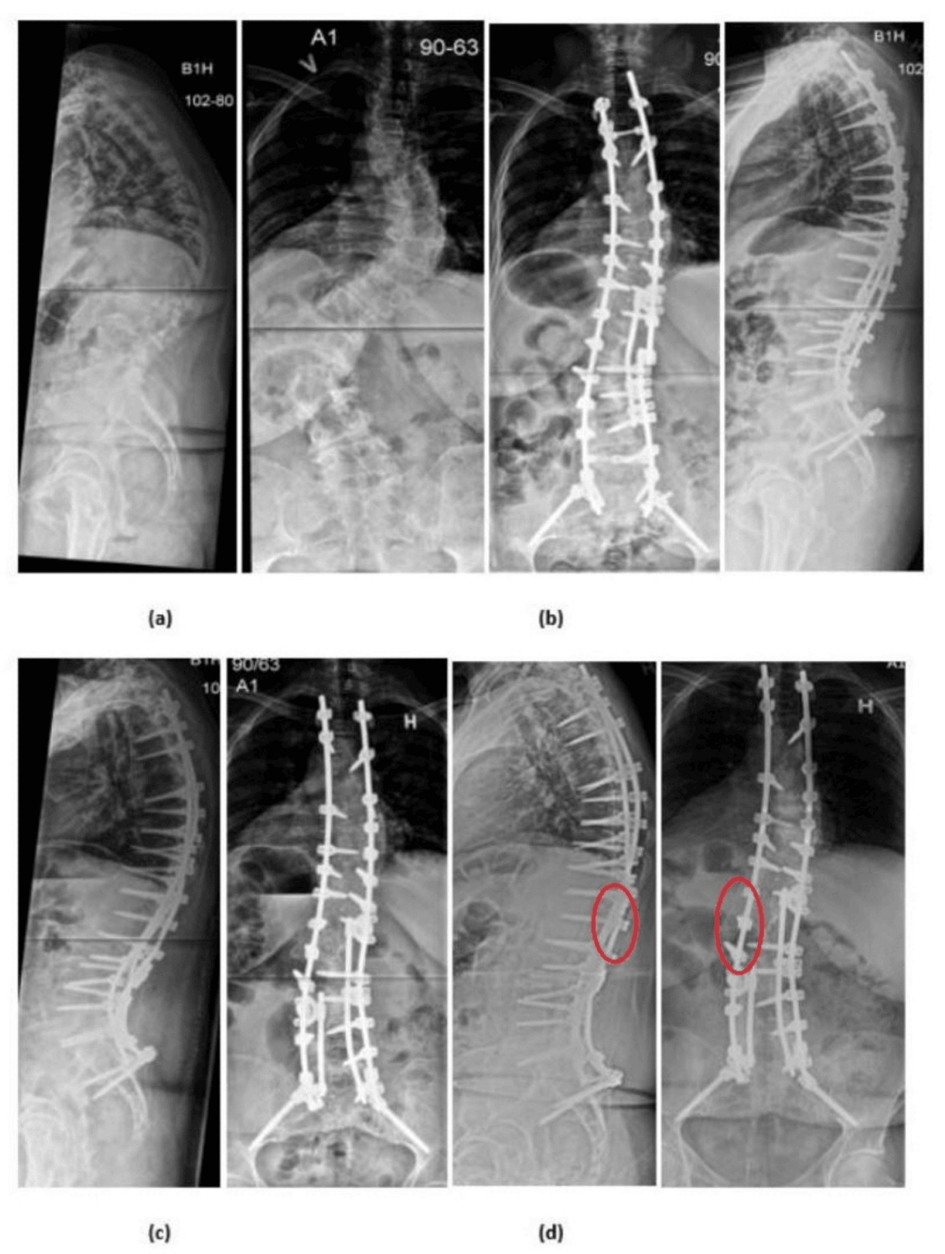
Case 2 A 53-year-old female was referred due to untreated thoracolumbar deformity known since childhood and progressing low back pain. She presented with kyphoscoliosis and a subjective feeling of positive sagittal imbalance (a). She underwent posterior instrumentation from Th2 to the pelvis with no supplemental ALIF (b). At 282 days postoperatively, the patient experienced acute onset of back pain and radiologically verified rod breakage due to pseudarthrosis. She underwent revision surgery with change of the rods and revision of the pseudarthrosis (c). At follow-up, the patient presented without pain and had returned to full-time employment. Radiographs showed rod breakage at L1, but with solid fusion and no loss of sagittal alignment, and thus was not scheduled for further revision (d). ALIF: anterior lumbar interbody fusion
